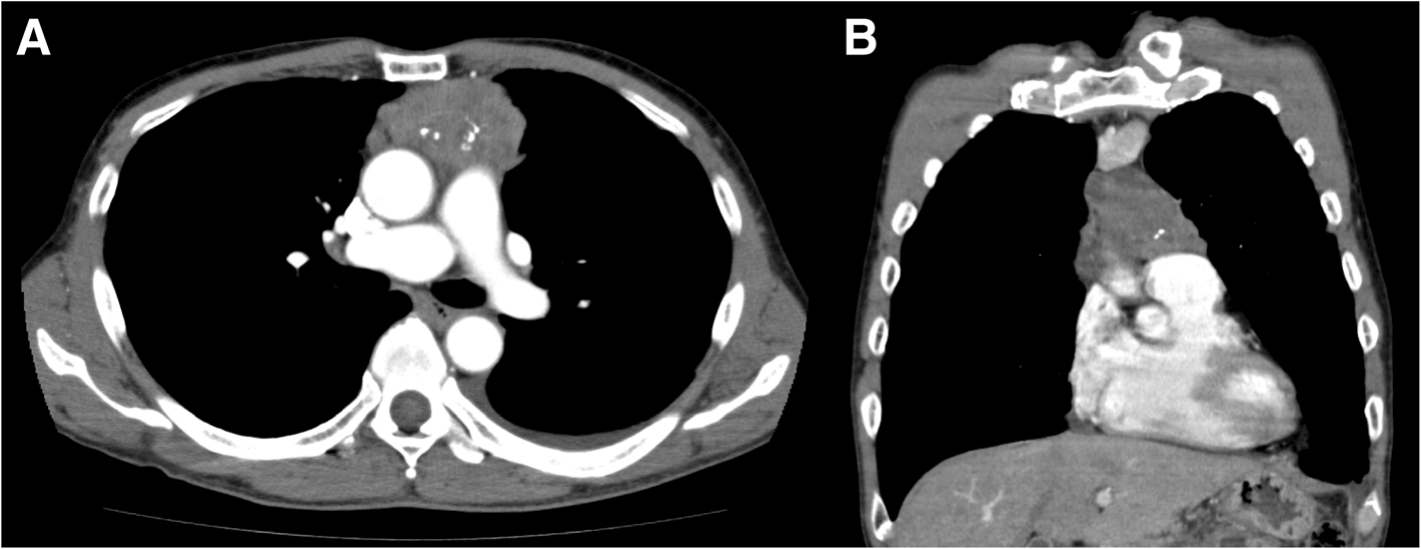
Computed tomography imaging of the chest at diagnosis. Transverse (a) or coronal (b) planes in a mediastinal window
